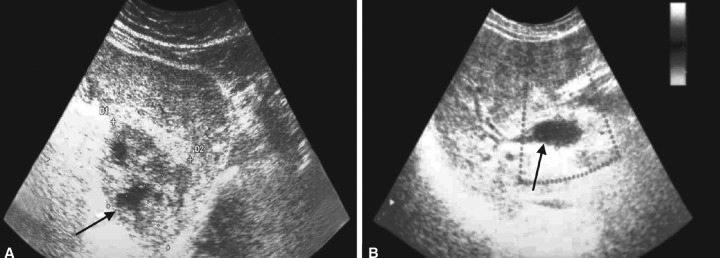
Abdominal ultrasound: (A) Barely separated hypoechoic lesion in the left hepatic lobe (arrow): 55.7 × 45 mm, with 2 echo-negative areas with diameters of 14 and 9 mm, with hyperechoic surroundings on the peripheral side of the left hepatic lobe; (B) the presence of pneumobilia in the left hepatic lobe. The common bile duct (CBD) dilated in the hilus (arrow) with a diameter of 14.9 mm and left lobular accentuation
